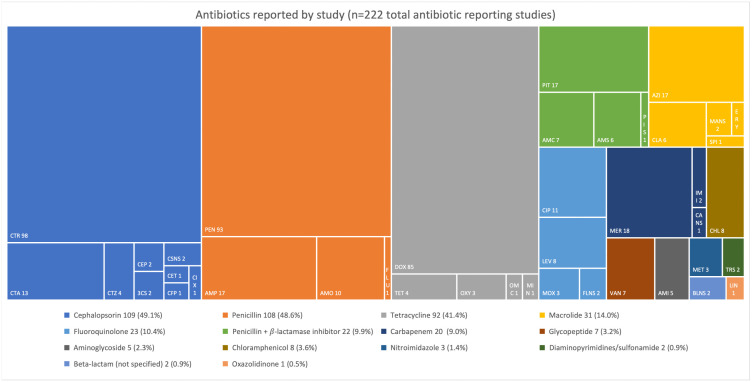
Treemap plot of antibiotics reported by studies. Abbreviations follow European Committee on Antimicrobial Susceptibility Testing nomenclature except for *: CTR Ceftiaxone, CTA Cefotaxime, CTZ Ceftazidime, CEP Cefepime, CET Cefalothin, *CFP Cefoperzone, CIX Cefixime, *3CS Third generation cephalosporin (not specified), *CSNS Cephalosporin (not specified), PEN Penicllin, AMO Amoxicillin, FLU Flucloxacillin, AMP Ampicillin, DOX Doxycyline, TET tetracycline, OXY Oxytetracycline, *OMC Omadacycline, MIN Minocycline, AZI Azithromycin, CLA Clarithromycin, ERY Erythromycin, *SPI Spiramycin, *MANS Macrolide (not specified), CIP Ciprofloxacin, LEV Levofloxacin, MOX Moxifloxacin, *FLNS Fluoroquinolone not specified, PIT Piperacillin-Tazobactam, AMC Amoxicillin-Clavulanic Acid, AMS Ampicllin-Sulbactam, *PIS Piperacillin-Sulbactam, MER Meropenem, IMI Imipenem, *CANS Carbapenem not specified, VAN Vancomycin, AMI Amikacin, CHL Chloramphenicol, MET Metronidazole, TRS Trimethoprim/sulfamethoxazole, *BLSN β-Lactam not specified, LIN Linezolid [19].
